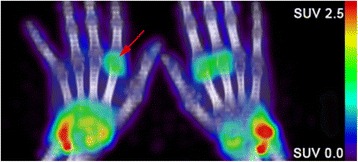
Example of a ^89^Zr-rituximab positron emission tomography (PET) image of the wrists/hands of a patient with rheumatoid arthritis who had multiple PET-positive joints. *Red arrow* represents one PET-positive joint (*green/yellow*). *SUV* standardized uptake values
